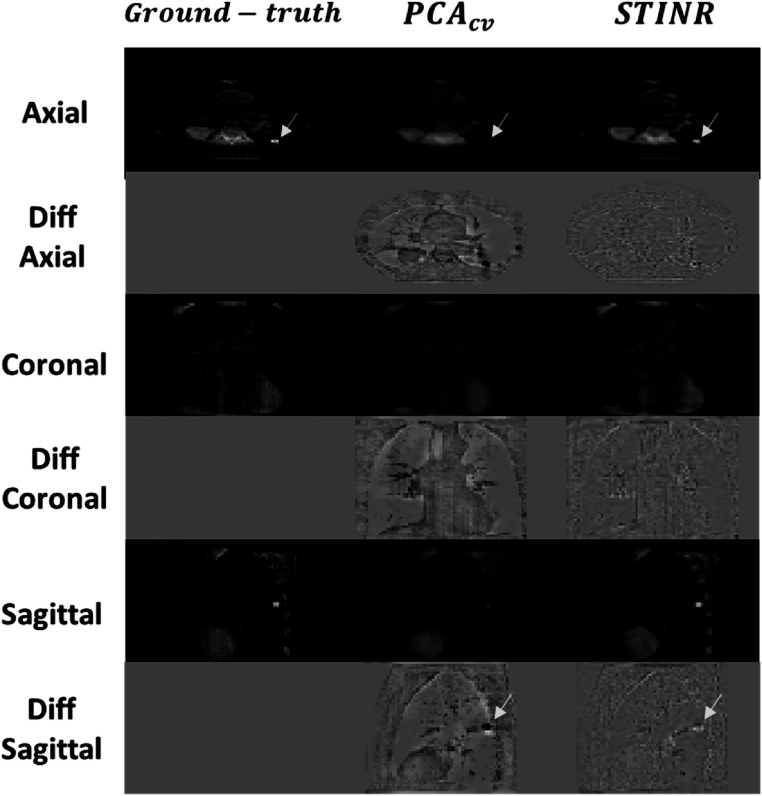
Comparison between the reconstructed CBCT volumes by $PC{A}_{cv}$ and STINR, and the ‘ground-truth’ CBCT
